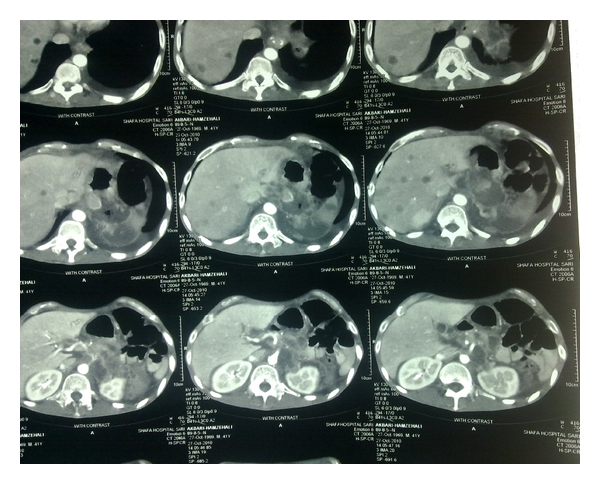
Abdominal CT Scan with contrast.
